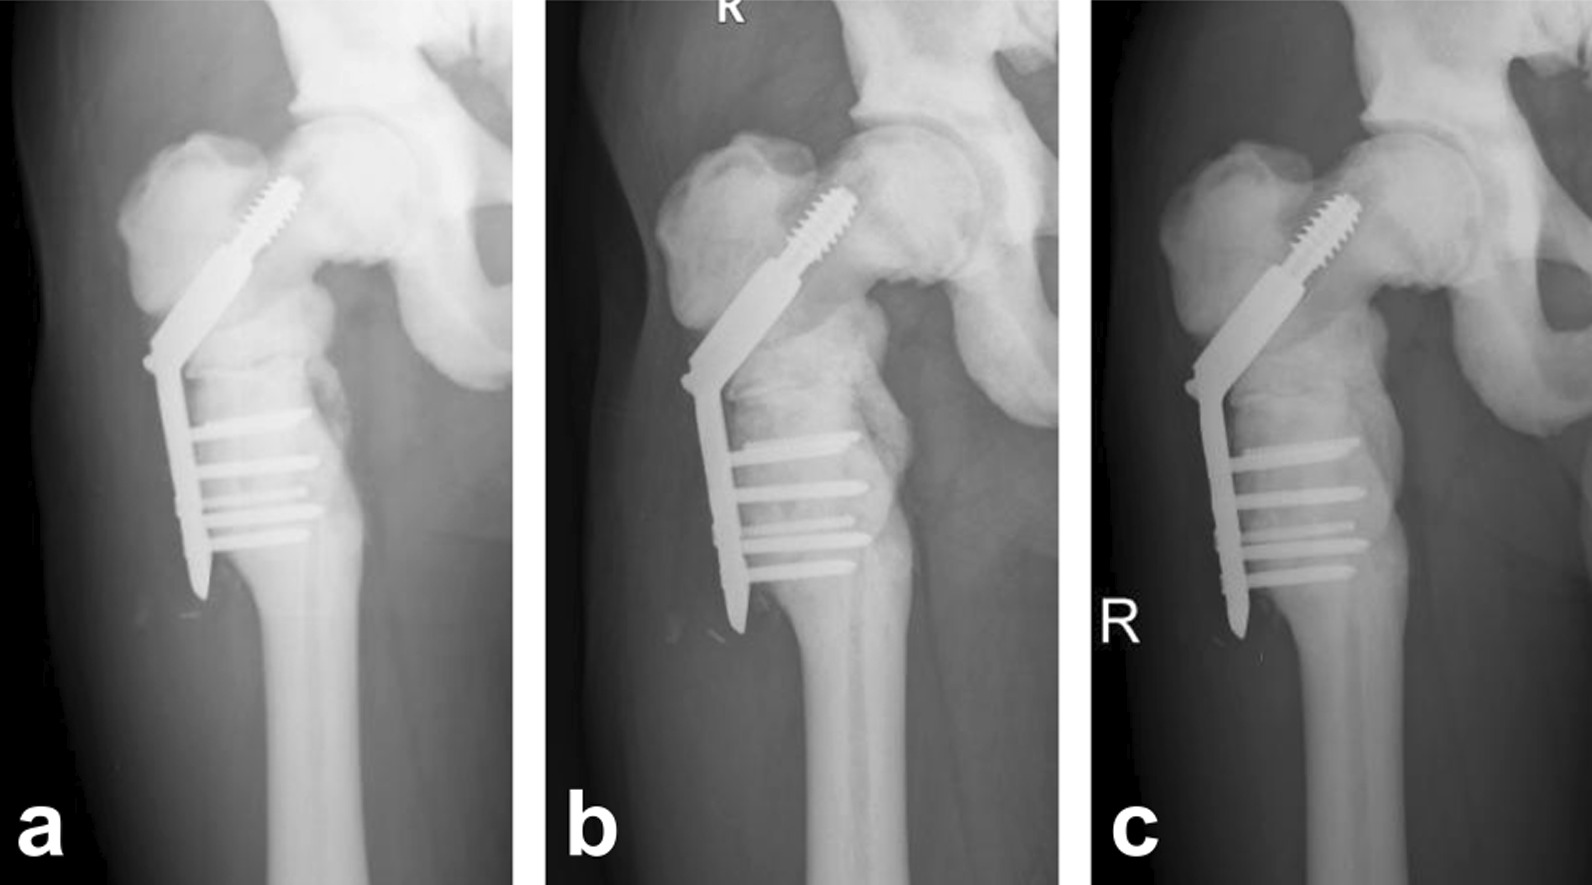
X-rays. a 3 months after revision. b 6 months after the revision. c 12 months after revision
